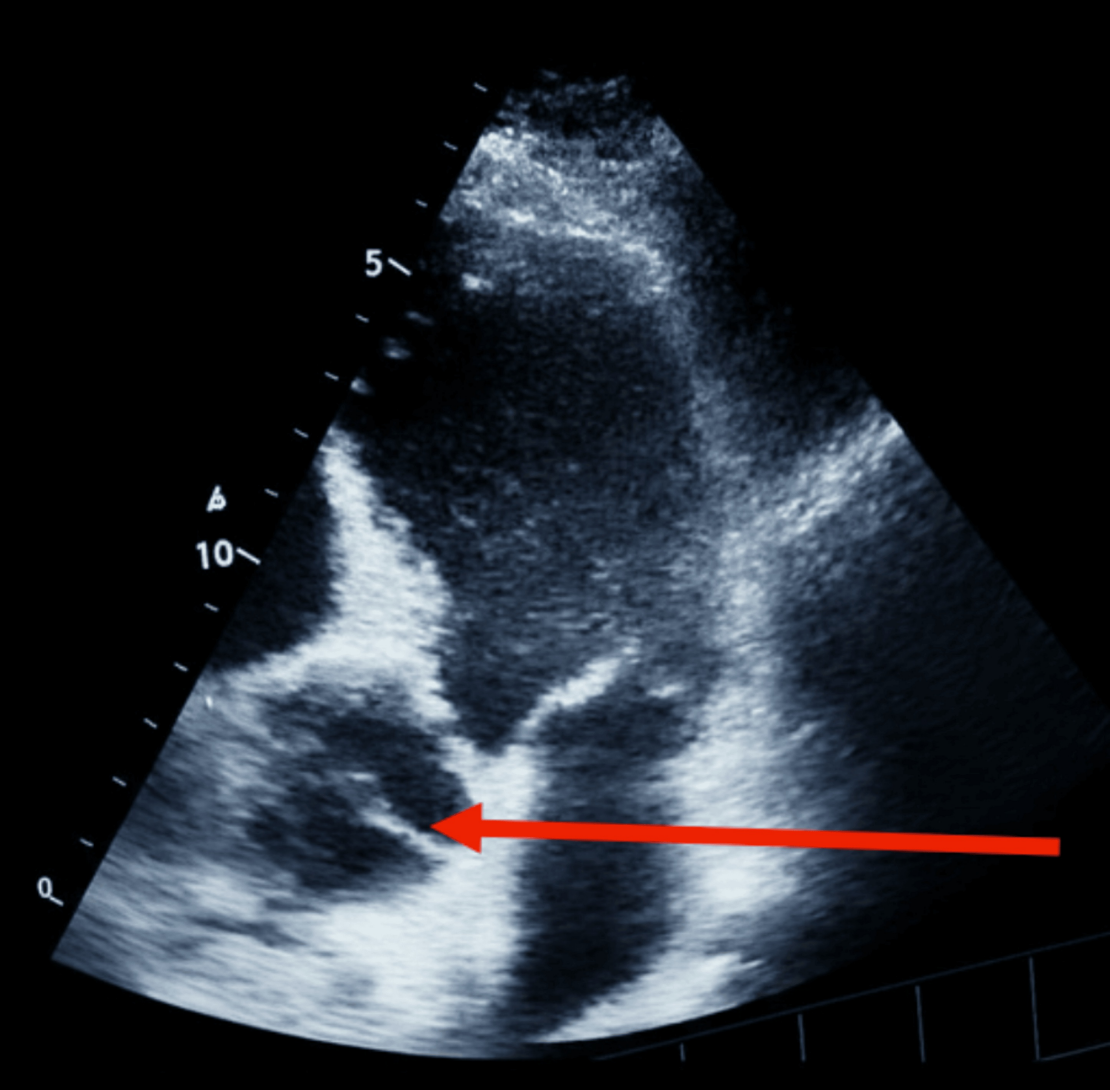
Two-dimensional apical four-chamber transthoracic echocardiogram showing a dilated ascending aorta and a dissection flap (arrow)
